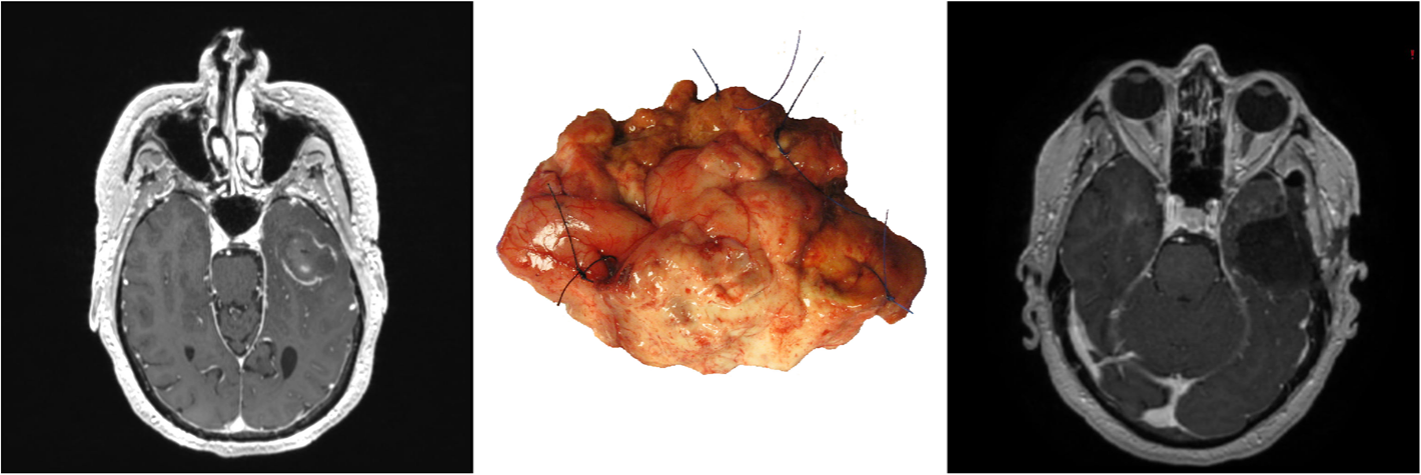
Magnetic resonance (MR)-imaging and pathologic findings at resection of left temporal laser-induced thermal therapy treated glioblastoma multiforme (GBM). Preoperative (before resection) T1-weighted post-contrast MR-imaging in the axial *(Left*) plane demonstrates enhancing necrotic region of treatment/tumor. (*Middle*) *En bloc* resection of the enhancing GBM/treatment site and surrounding tumor-infiltrated tissue (6.5 cm anteroposterior and 4.4 cm mediolateral dimension) was performed. Postoperative T1-weighted post-contrast MR-imaging in the axial (*Right*) plane demonstrates complete resection of the enhancing necrotic region of treatment/tumor
